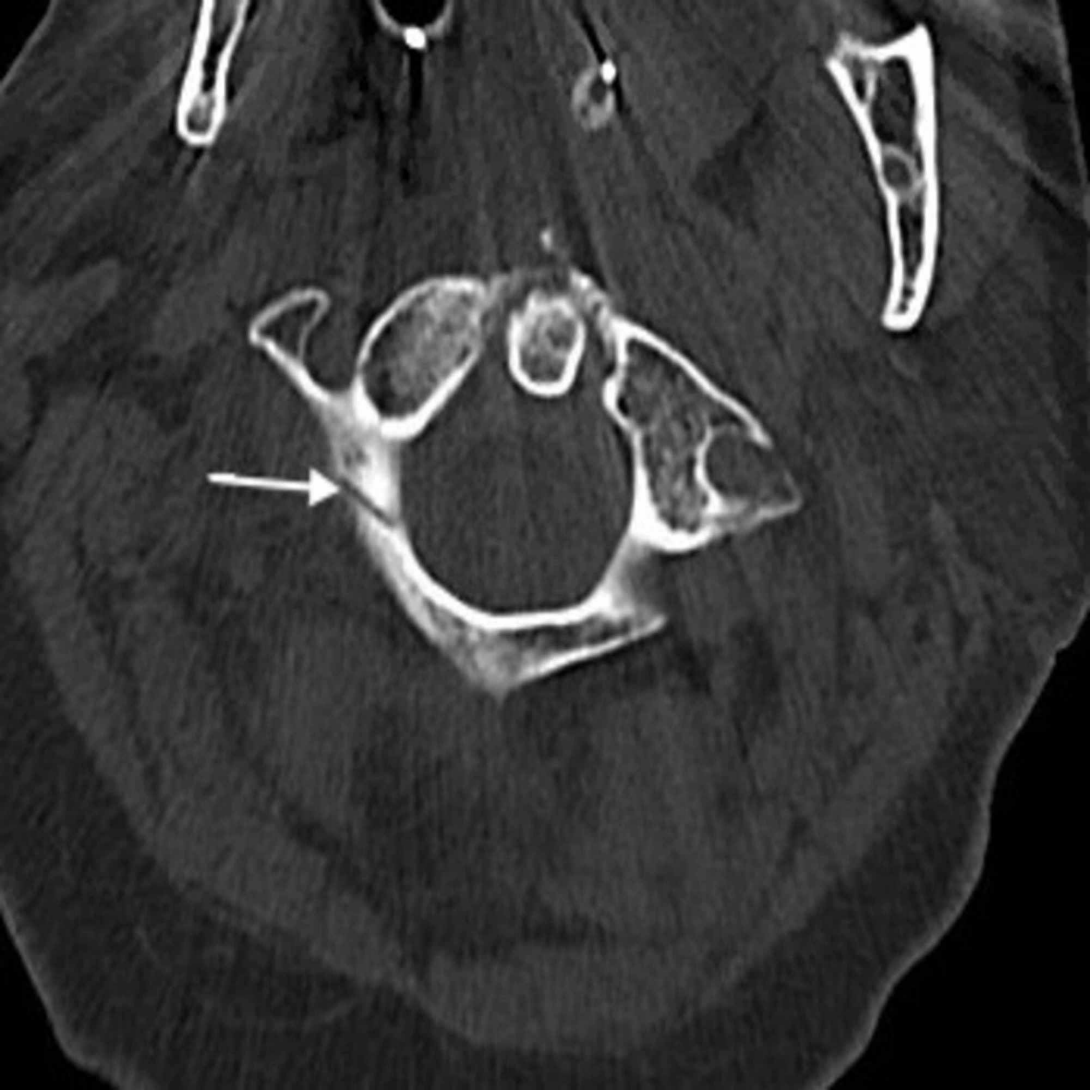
Axial CT of the cervical spine showing C1 posterior arch fracture. There is an acute non-displaced C1 arch fracture with bilateral transverse foramen involvement without displacement.
